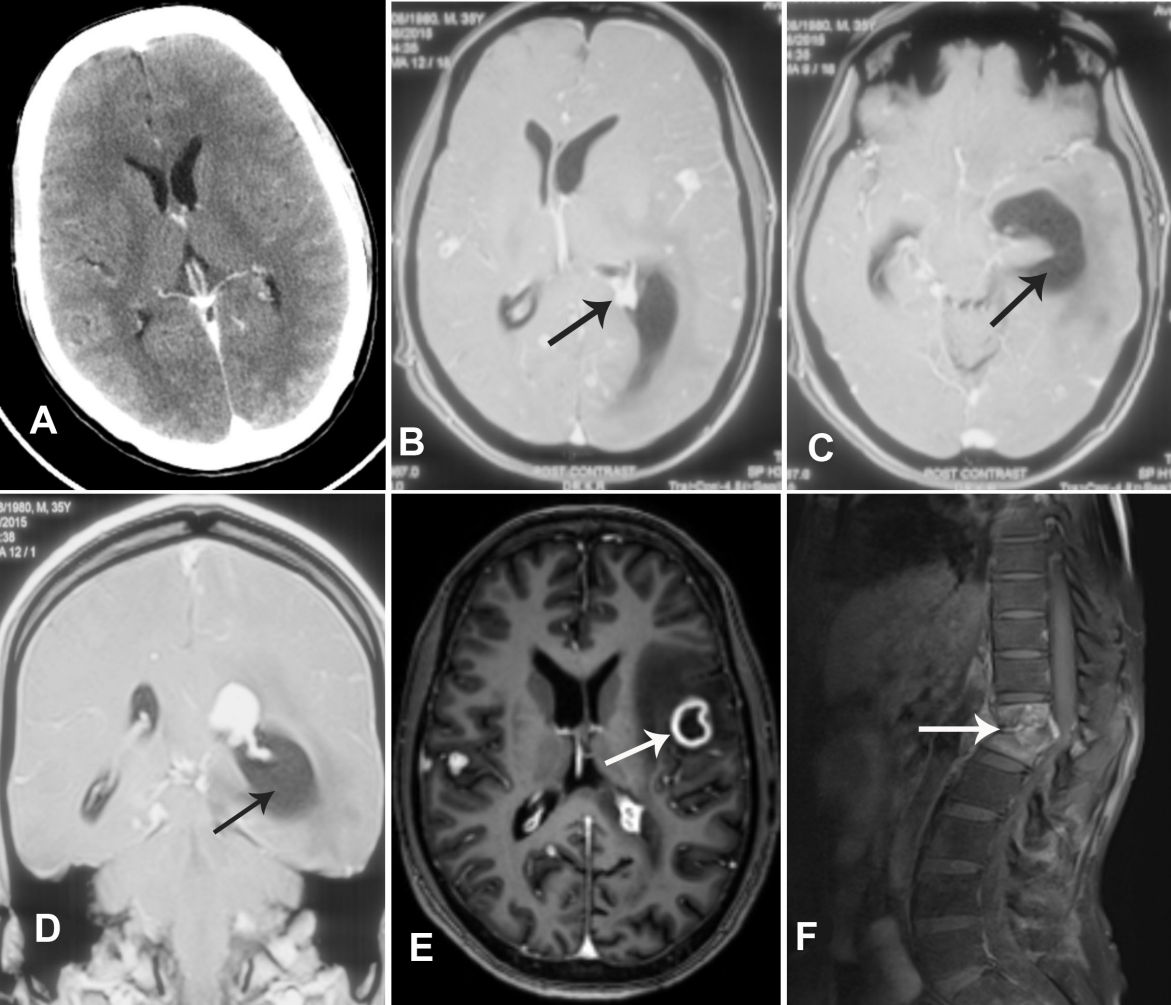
A computer Tomography carried out at A) diagnosis of tubercular did not show any lesion. B) Post gadolinium contrast enhanced MRI at time of presentation revealing a left atrial enhancing lesion causing loculated temporal horn (arrow shows). C & D) Loculated temporal horn caused by a lesion in left atrium of lateral ventricle (arrow shows). E) Post gadolinium contrast enhanced MRI revealing left frontal opercular ring enhancing lesion (arrow shows). F) MRI of spine showing D12-L1 vertebral collapse with para-spinal abscess enhancing on contrast. (arrow shows)
